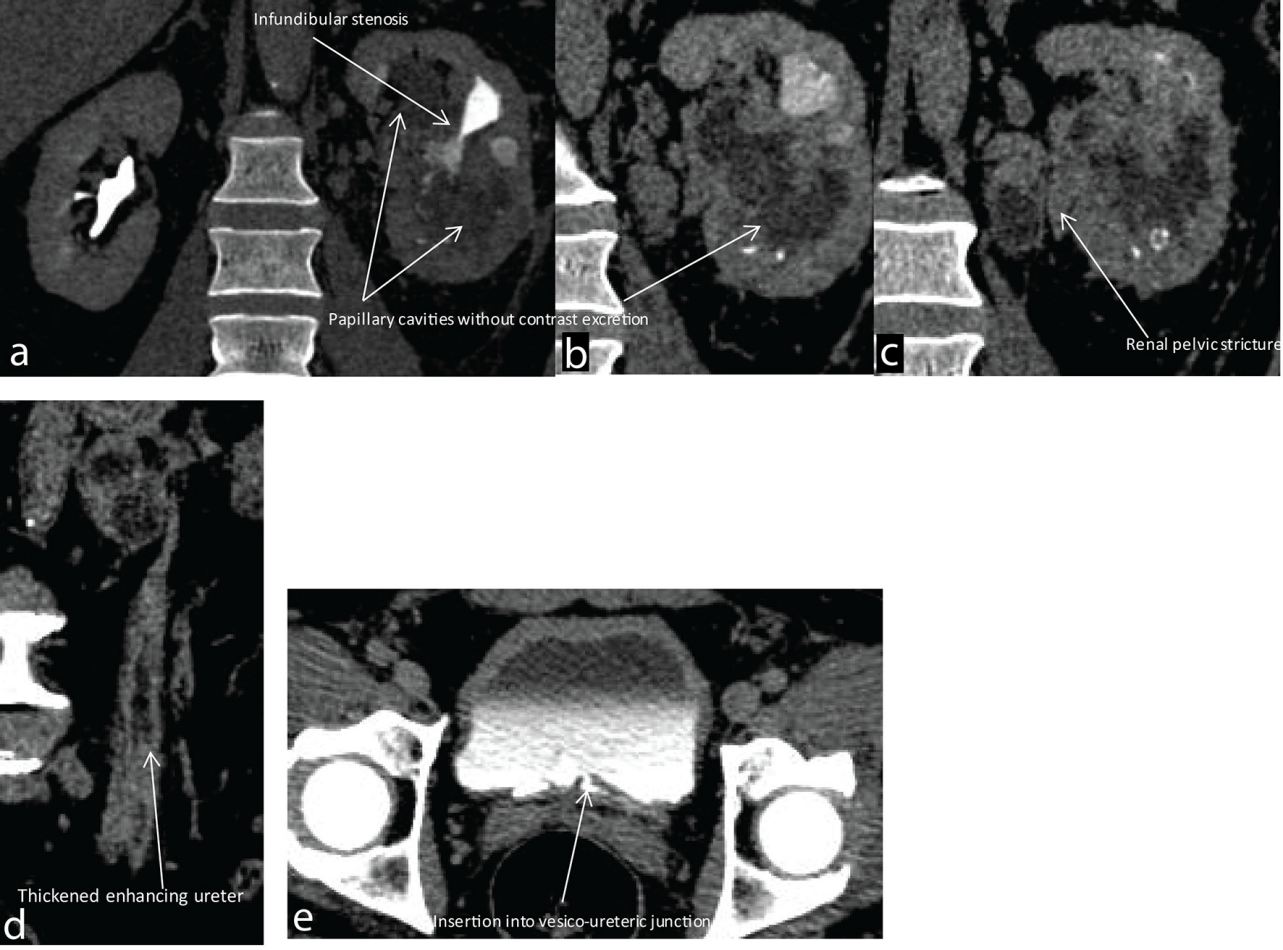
(a–d) Coronal reformats of CT urogram images showing (a,b) papillary cavities with distorted calyces in the upper and lower poles without opacification by contrast. Infundibular stenosis with a dilated triangular-shaped calyx is seen suggesting a papillary cavity with excreting calyces or a papillary cavity showing communication with the calyceal system. (c) Also seen is the narrowed lumen of the renal pelvis with wall thickening suggesting a renal pelvic stricture. A necrotic hilar lymph node is also seen in these images. (d) Coronal reformat showing irregularly thickened enhancing walls of the ureter. (e) - -, The terminal ureter and the vesicoureteral junction shows wall thickening.
